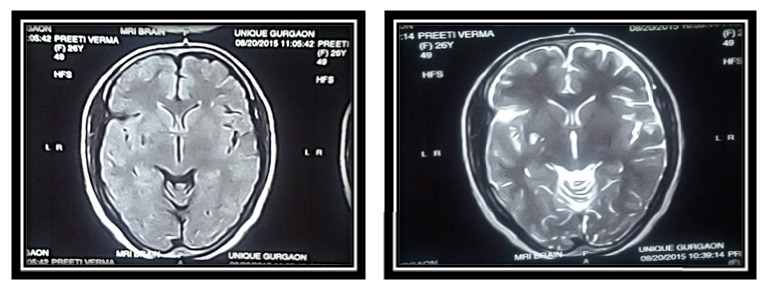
MRI brain scan reveals no focal pathology.
